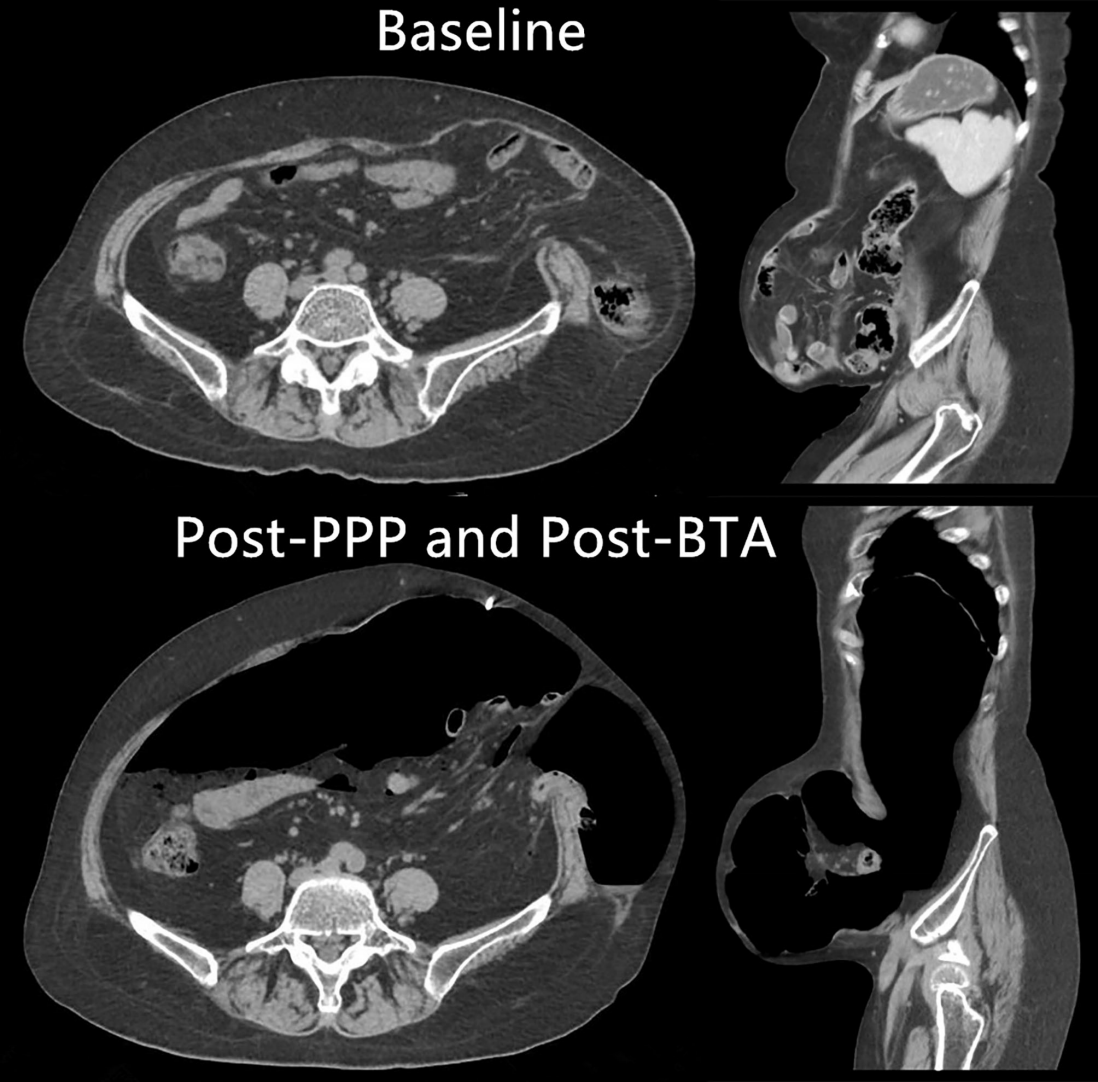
Abdominal CT picture of before and after PPP and BTA treatments demonstrating spontaneously reinstate the herniated contents.
